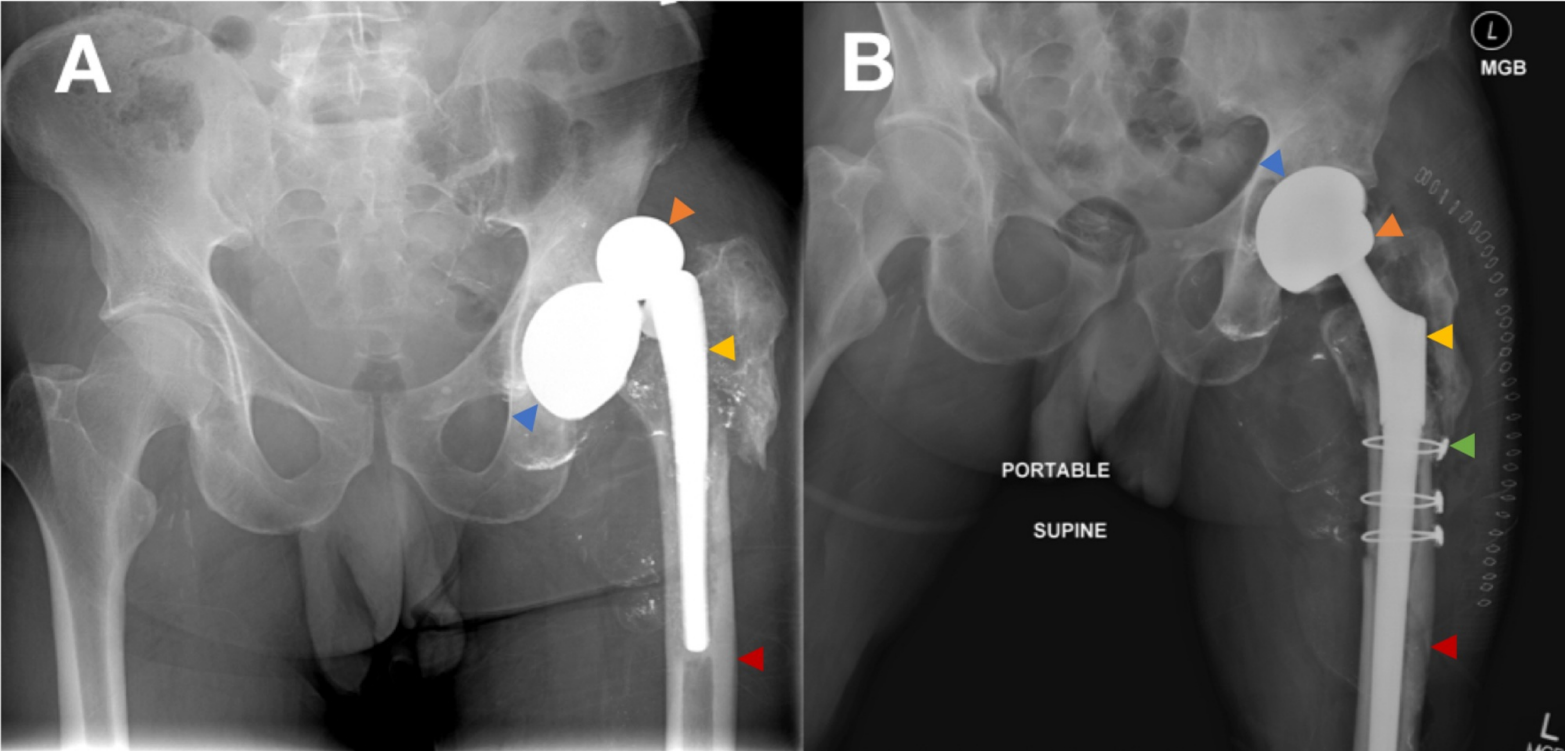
Representative Staged Radiographs for Revision Images A and B display radiographs of a patient who underwent left revision THA during the study period. Image A displays a preoperative AP pelvis radiograph prior to first revision THA. Image B displays an AP pelvis radiograph nine days status post first revision THA. The blue arrows demonstrate the acetabular cup. The orange arrows demonstrate the femoral head. The yellow arrows demonstrate the femoral component. The red arrows demonstrate the femur. The green arrow demonstrates the cerclage wires used in revision. THA: total hip arthroplasty; AP: anteroposterior
